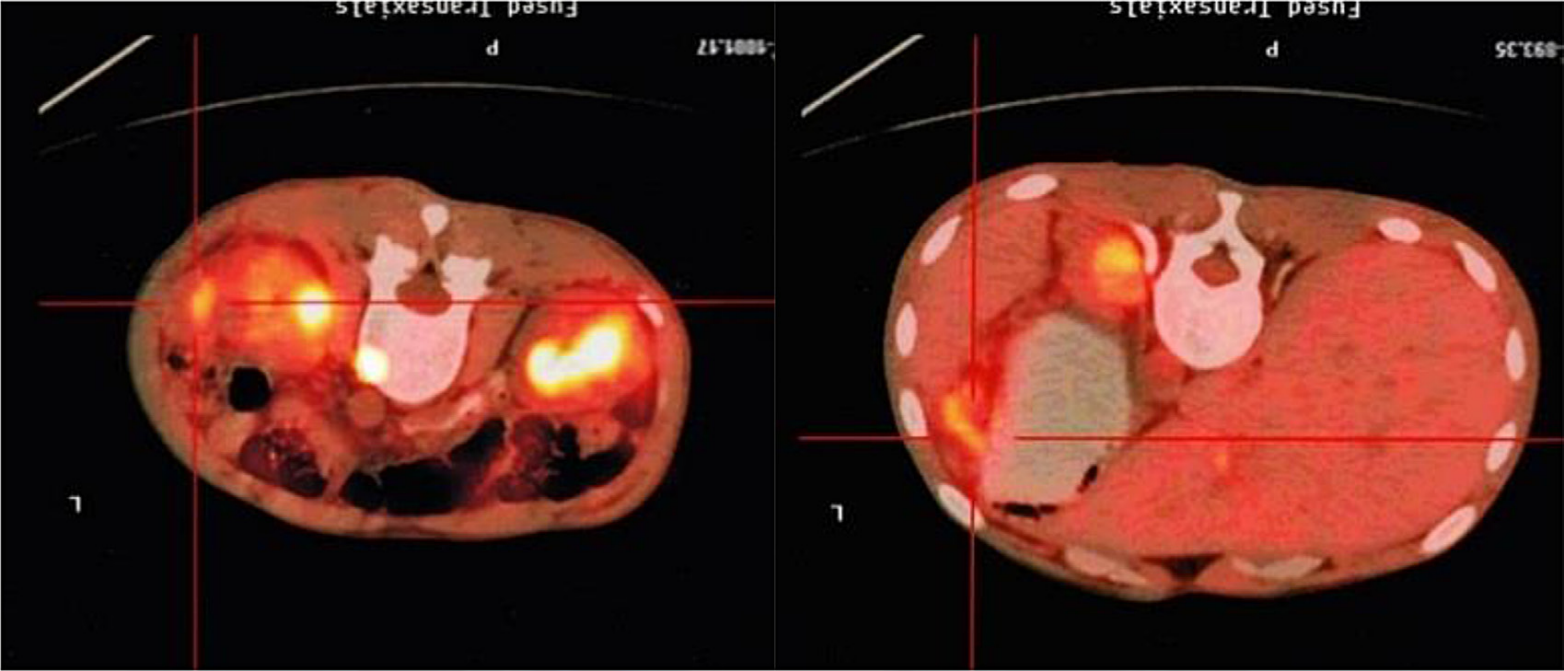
PET-CT image of a patient (male, ETO+, AML) showing widespread violations of granulocytic sarcoma
